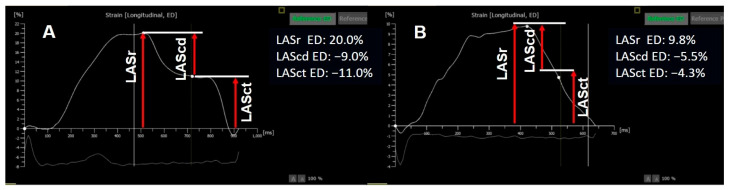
A 65-year-old female patient, with a 12-year diagnosis of chronic lymphocytic leukemia and unfavourable evolution under multiple antineoplastic therapies, some with cardiotoxic potential, with relapse of the disease. She was referred for baseline cardiologic evaluation due to increased risk of cardiotoxicity. Following the assessment, she was started on the second-generation Bruton’s tyrosine kinase inhibitor, acalabrutinib. (A) Baseline echocardiographic evaluation showed normal phasic components of left atrial strain but with reduced LASr (20%). (B) One month after initiating acalabrutinib, the patient developed paroxysmal atrial fibrillation. Reevaluation of her echocardiogram indicated a decline in all phasic components of left atrial strain, while her left atrial volume remained unchanged. LASr: LA strain reservoir; LAScd: LA strain conduit; LASct: LA strain contractile.
